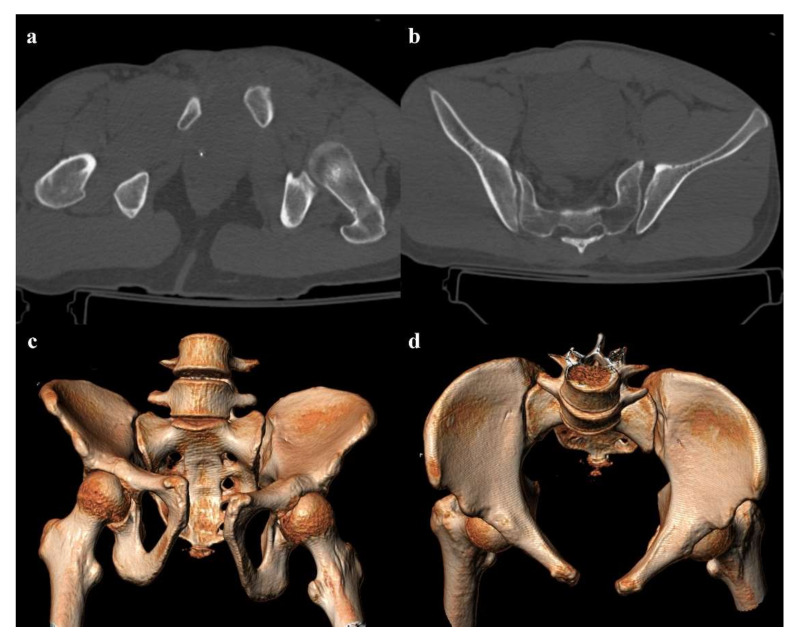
Anterior–posterior compression fracture, type 2. Axial CT images (a,b) show a pubic symphysis diastasis of 3.2 cm, and an anterior sacroiliac joint diastasis on the left side. Three-dimensional volume-rendering CT reconstructions in outlet and inlet views (c,d) confirm the findings.
